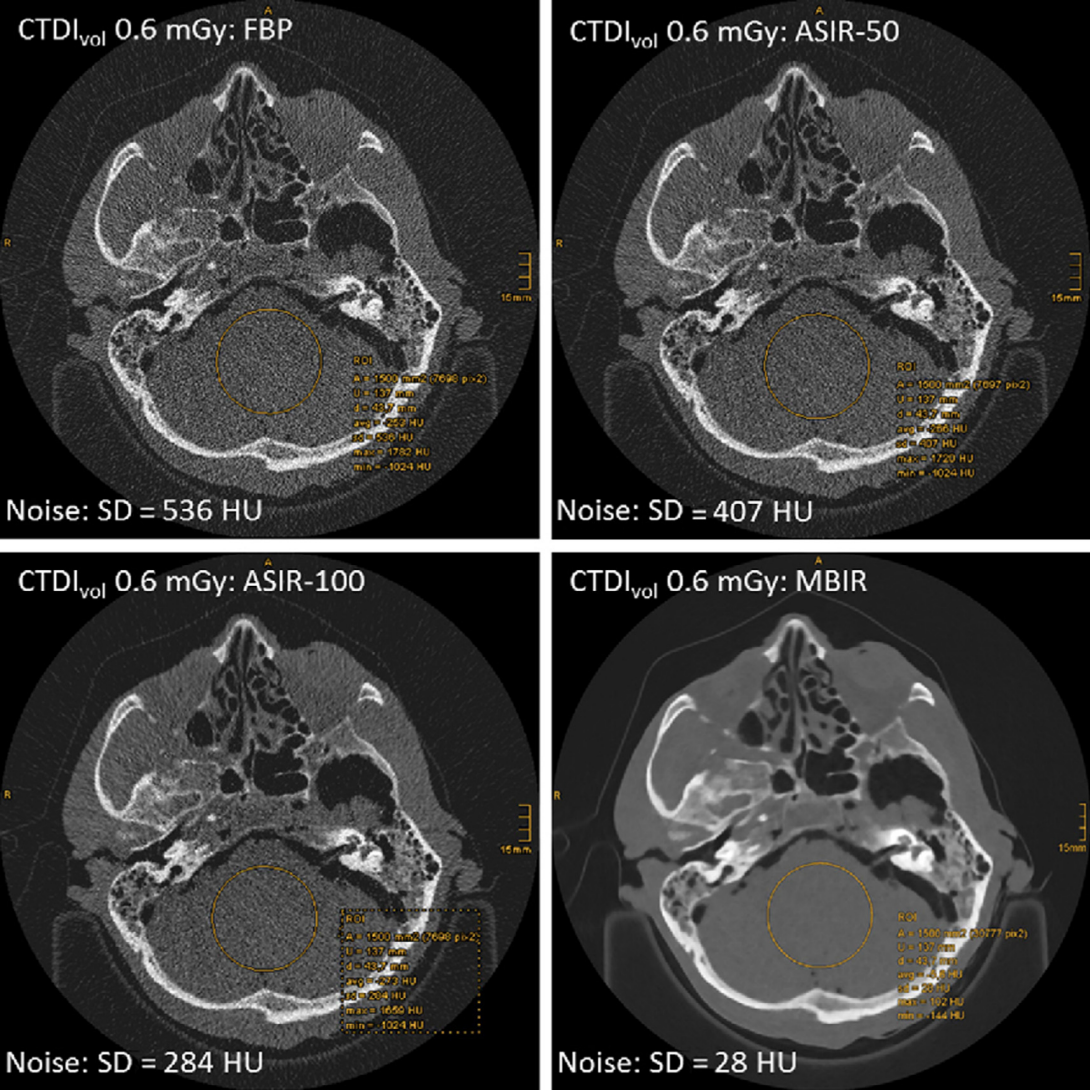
Noise measurement (SD) on axial slices of the mid‐facial region completed on CT with the lowest dose protocol. Results show the lowest noise with MBIR compared to FBP, ASIR‐50 and ASIR‐100 reconstructions.^37^ Permission was obtained to reproduce these images.
